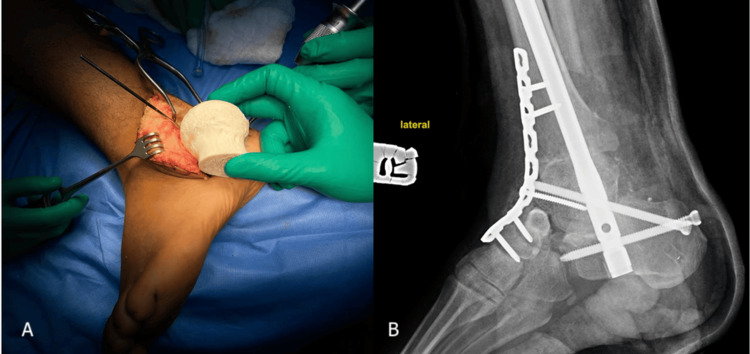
(A) Intraoperative and (B) X-ray image of the right foot showing femoral head allograft for talus defect reconstruction with tibiotalocalcaneal (TTC) fusion using a retrograde TTC nail with 3.5 LCD plate.
